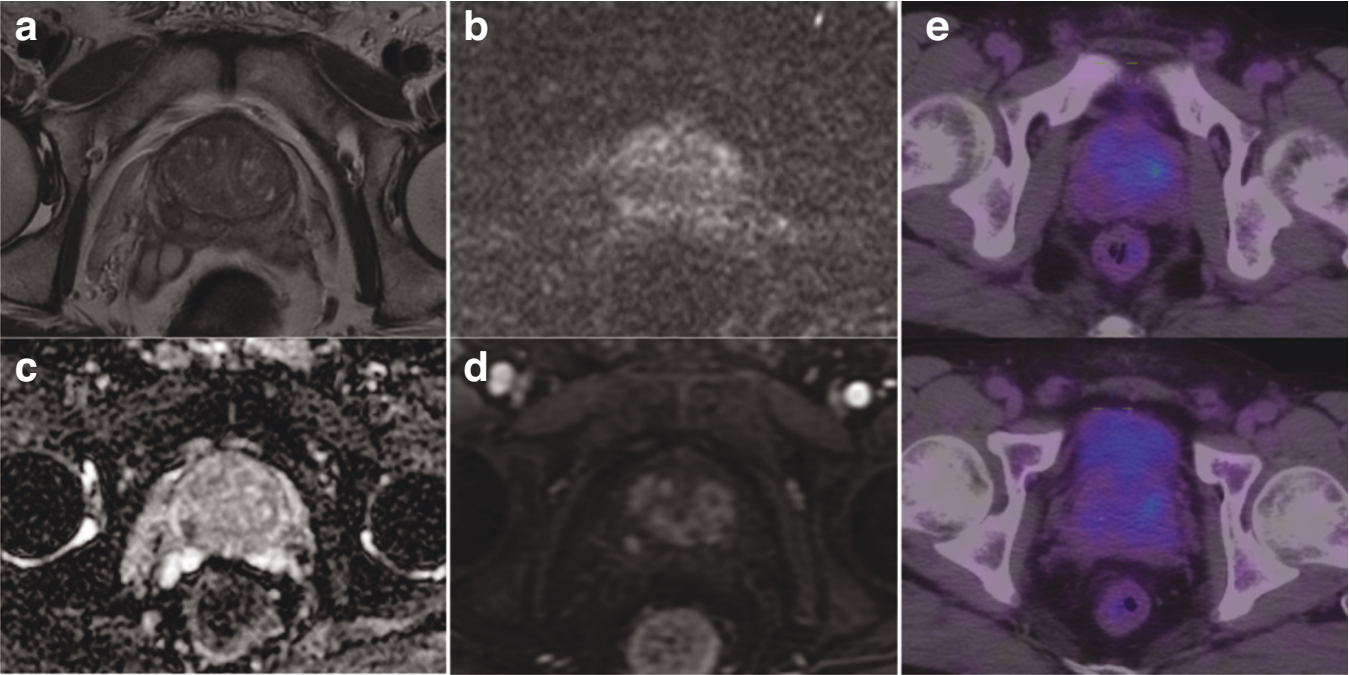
A PI-RADS 4 lesion on mpMRI was negative on ^18^F-PSMA-1007 PET/CT (SUVmax 6.1) and confirmed to be benign on biopsy. (a) T2-weighted image, (b) diffusion-weighted image, (c) apparent diffusion coefficient map, (d) dynamic-contrast enhanced image, and (e) ^18^F-PSMA-1007 PET/CT images.
